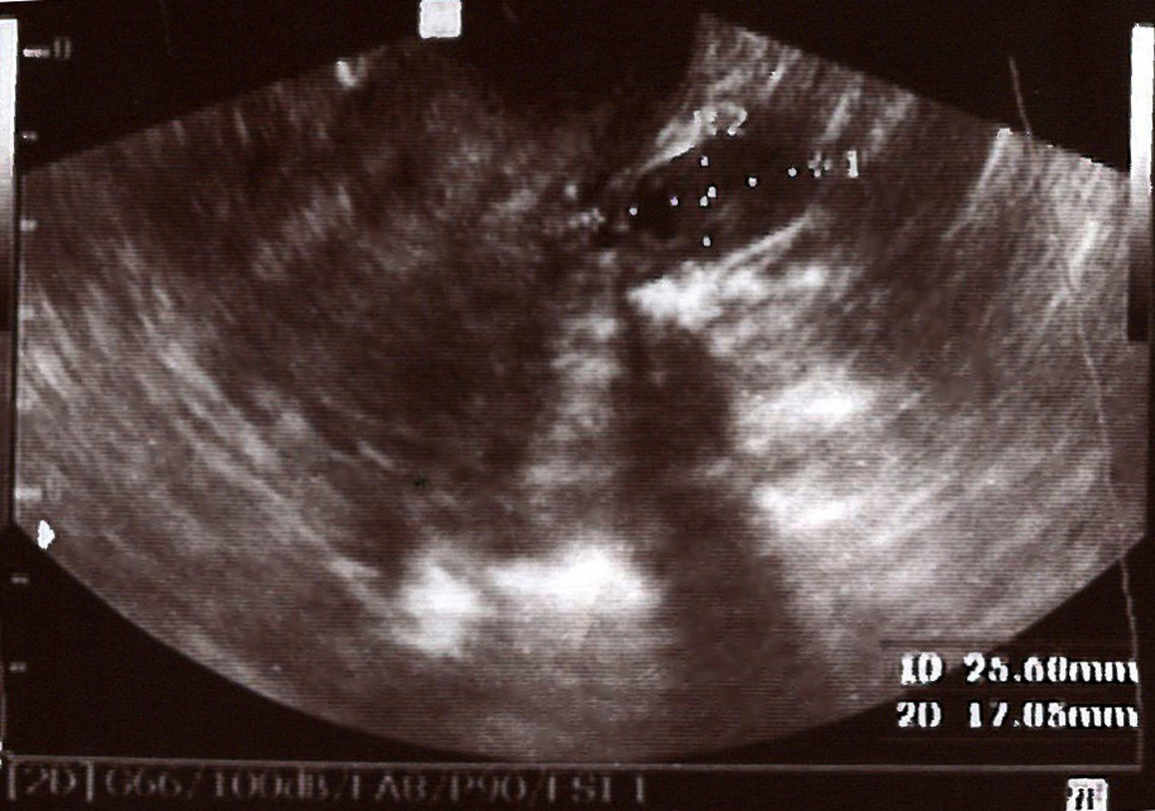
In the sonography after the radiotherapy, as can be seen, there is no trace of the lesion.
